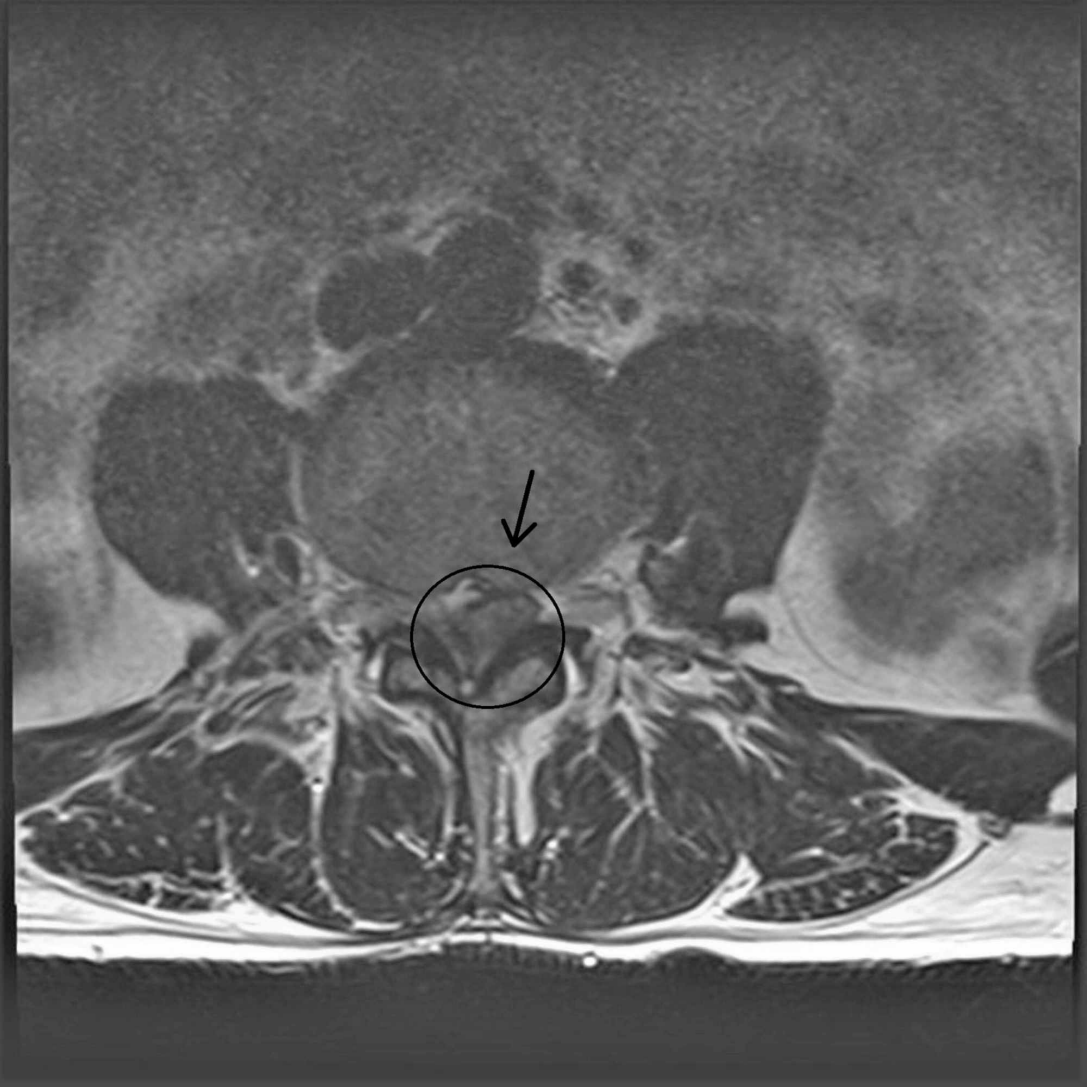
Axial image of MRI that shows disc fragment at the L4-L5 level.
